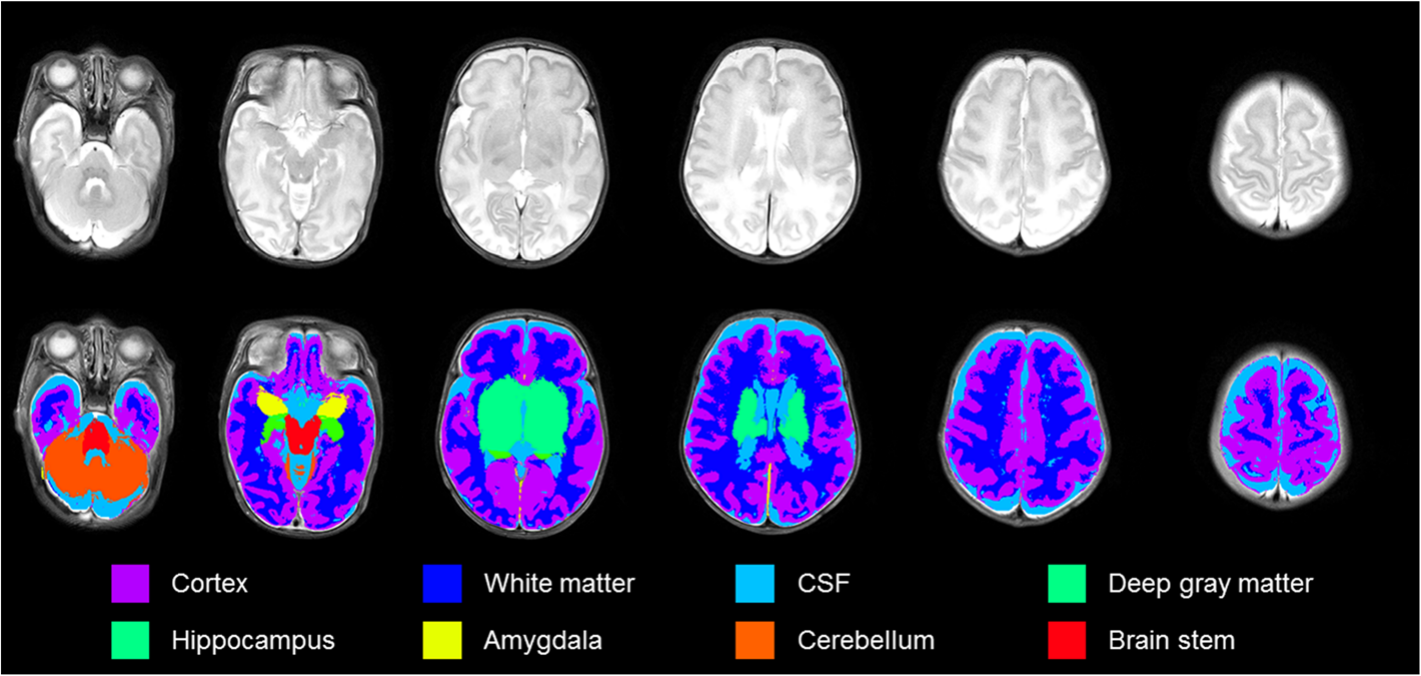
Example axial slices overlaid on T2-weighted images (1st row) and segmented into six brain morphological regions (2nd row). Subcortical gray matter includes hippocampus and amygdala. Abbreviations: CSF, cerebrospinal fluid
